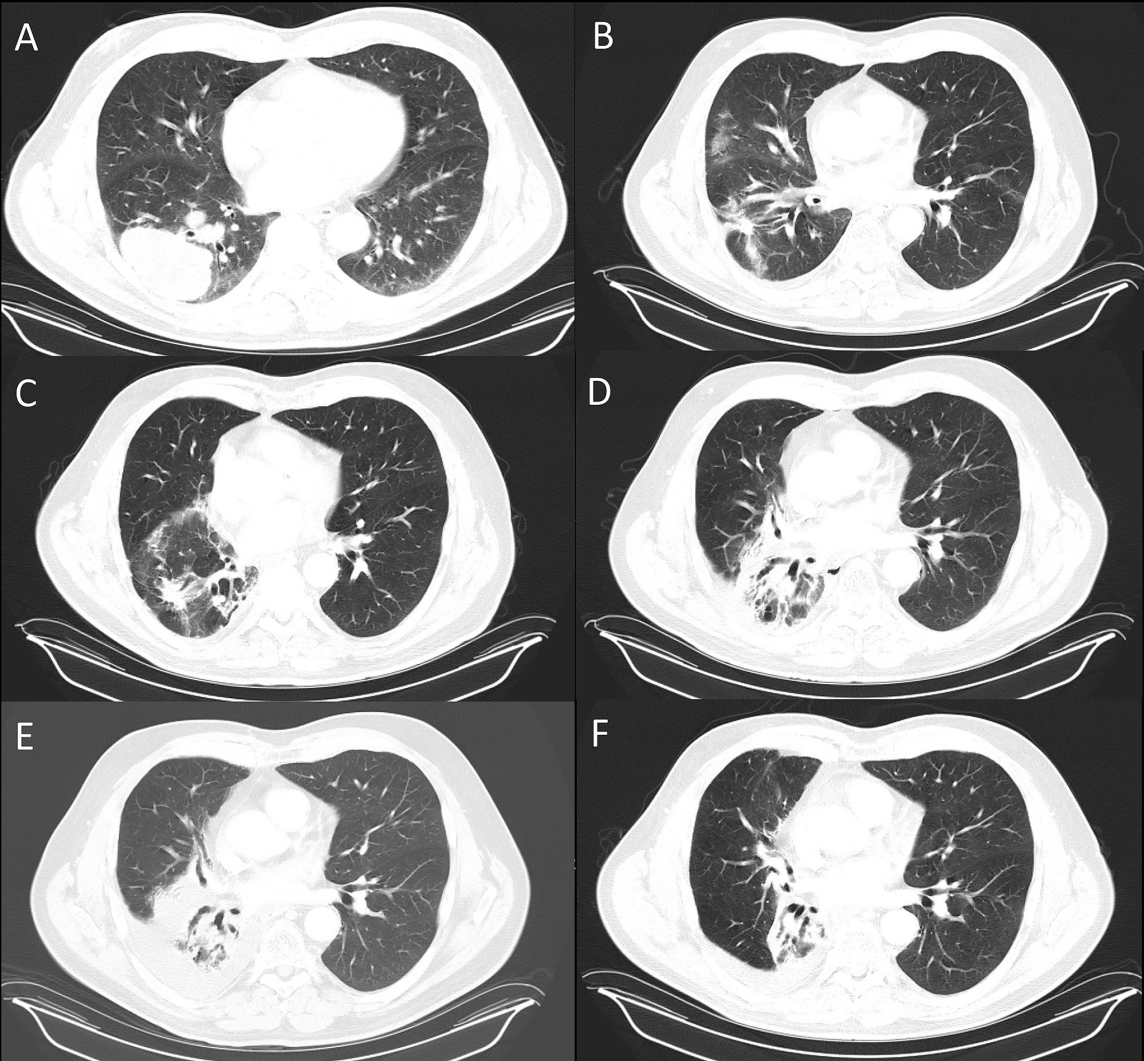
Serial CT’s of the chest shows resolution of lung pneumonitis with anabolic steroids. (A) Lung disease at presentation and after completion of definitive chemoRT at (B), 2 months (C), 5 months (D), 8 months (E), 11 months (F), 17 months. The androgen-HGH regimen was initiated after the 12 month scan, (E).
